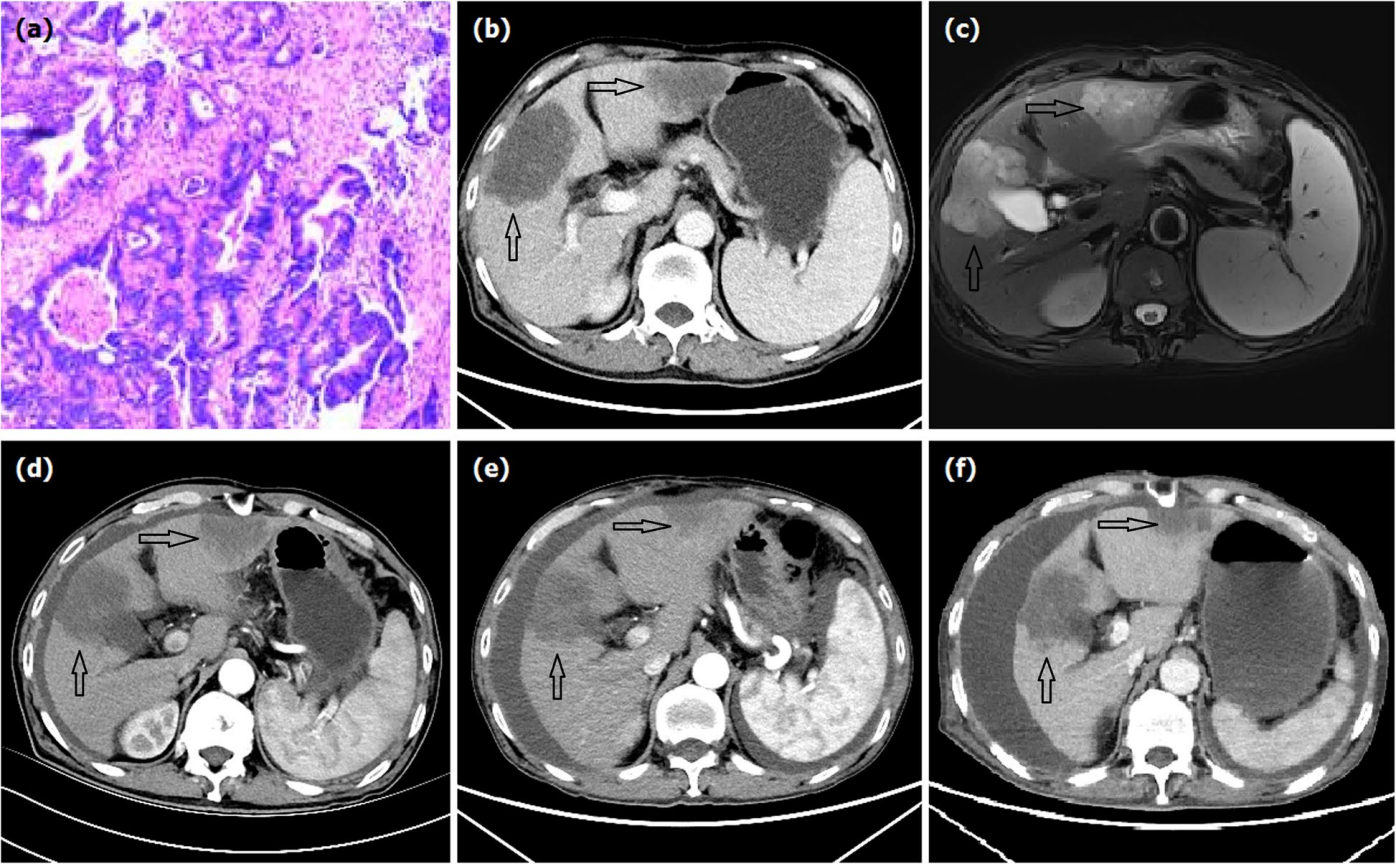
A 67-year male treated by CalliSpheres® beads for liver metastases from colonic cancers. a Colonic adenocarcinoma was confirmed pathologically. b, c Computed tomography and magnetic resonance imaging examination on admission revealed multiple intrahepatic metastases (arrows). d–f Computed tomography showed tumors decreased at 1, 3, and 6 months after DEB-TACE
